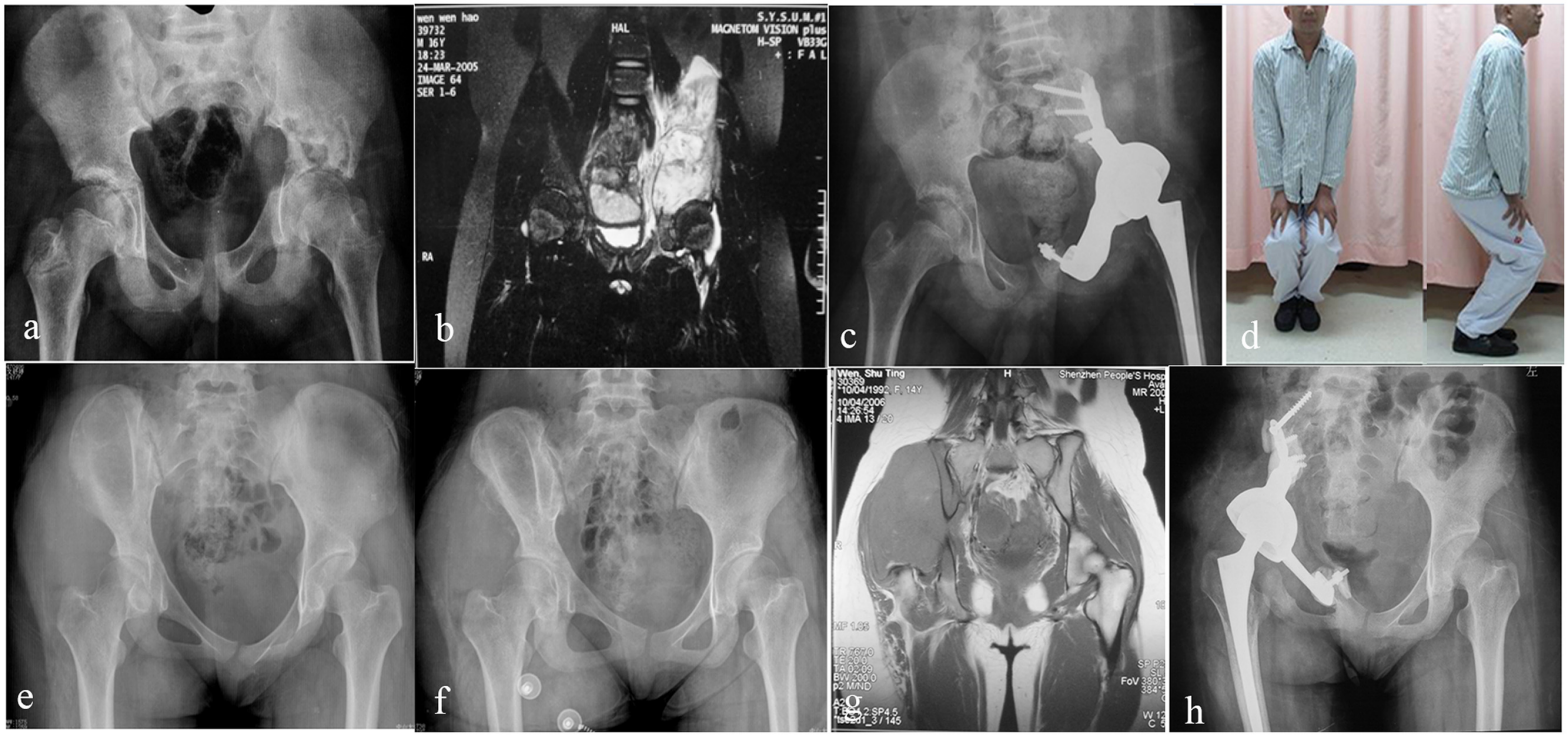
Patients follow-up. (a) and (b) Preoperative X-ray film and MRI of a patient with osteosarcoma involving section I/II of the left hemipelvis. (c) Postoperative X-ray film showing reconstruction with the modular hemipelvic endoprosthesis. (d) The function status of the hip joint of the same patient after reconstruction showing favorable extent of hip flexion. (e) Preoperative X-ray film of a patient with osteosarcoma involving section I/II of the right hemipelvis. (f) X-ray film after neo-adjuvant chemotherapy showing significantly enhanced osteogenesis inside the tumor. (g) Preoperative MRI. (h) Postoperative X-ray film showing reconstruction with the modular hemipelvic endoprosthesis.
